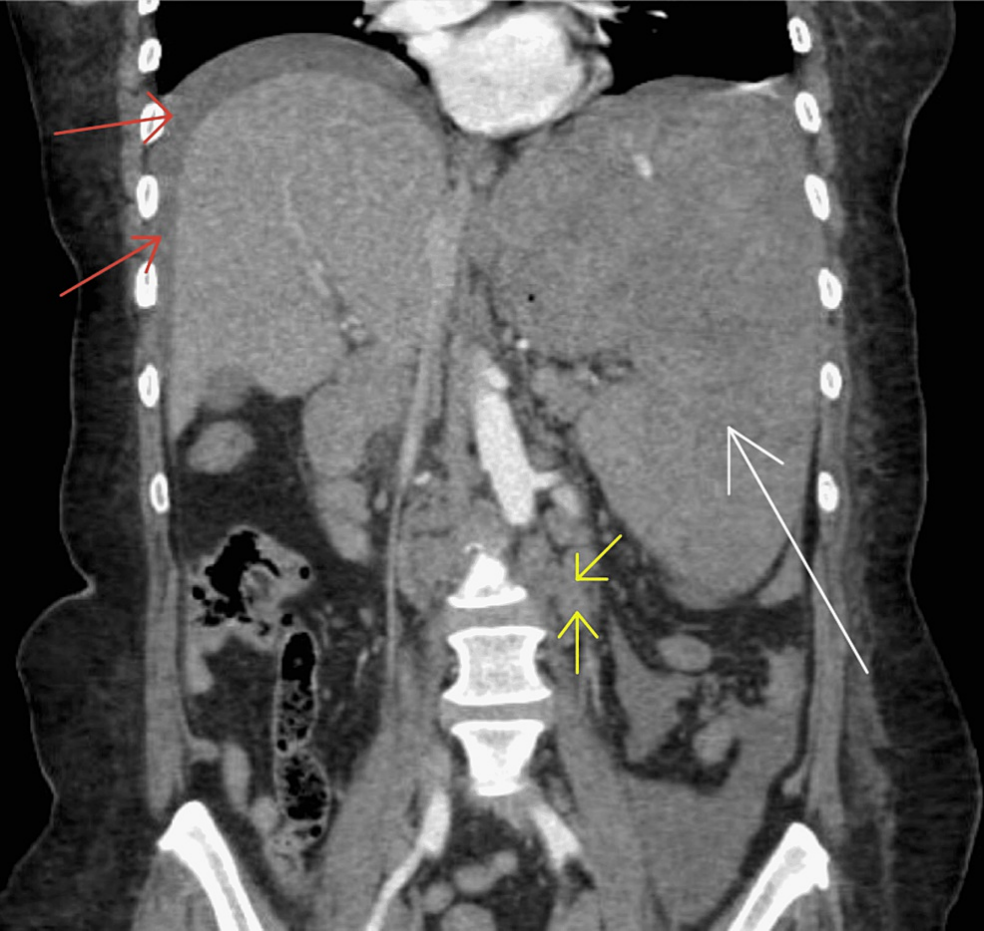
Coronal computerized tomography (CT) scan. Red arrows show gross hemoperitoneum. White arrow shows splenomegaly. Yellow arrows show enlarged abdominal lymph nodes.
